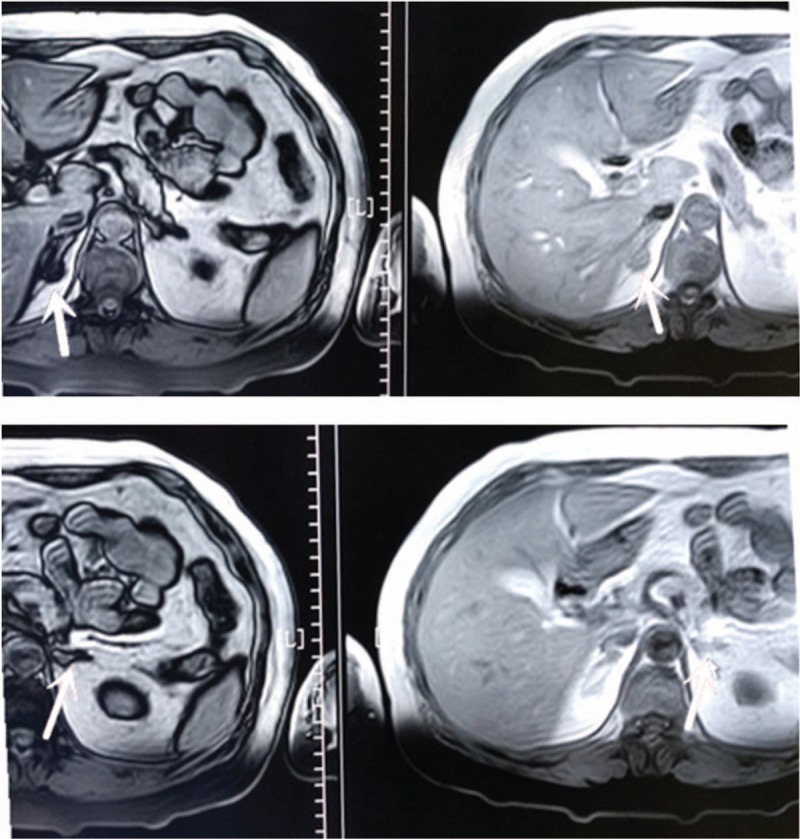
Bilateral adrenal magnetic resonance imaging (MRI) scan data showing the location of the bilateral adenomas.
